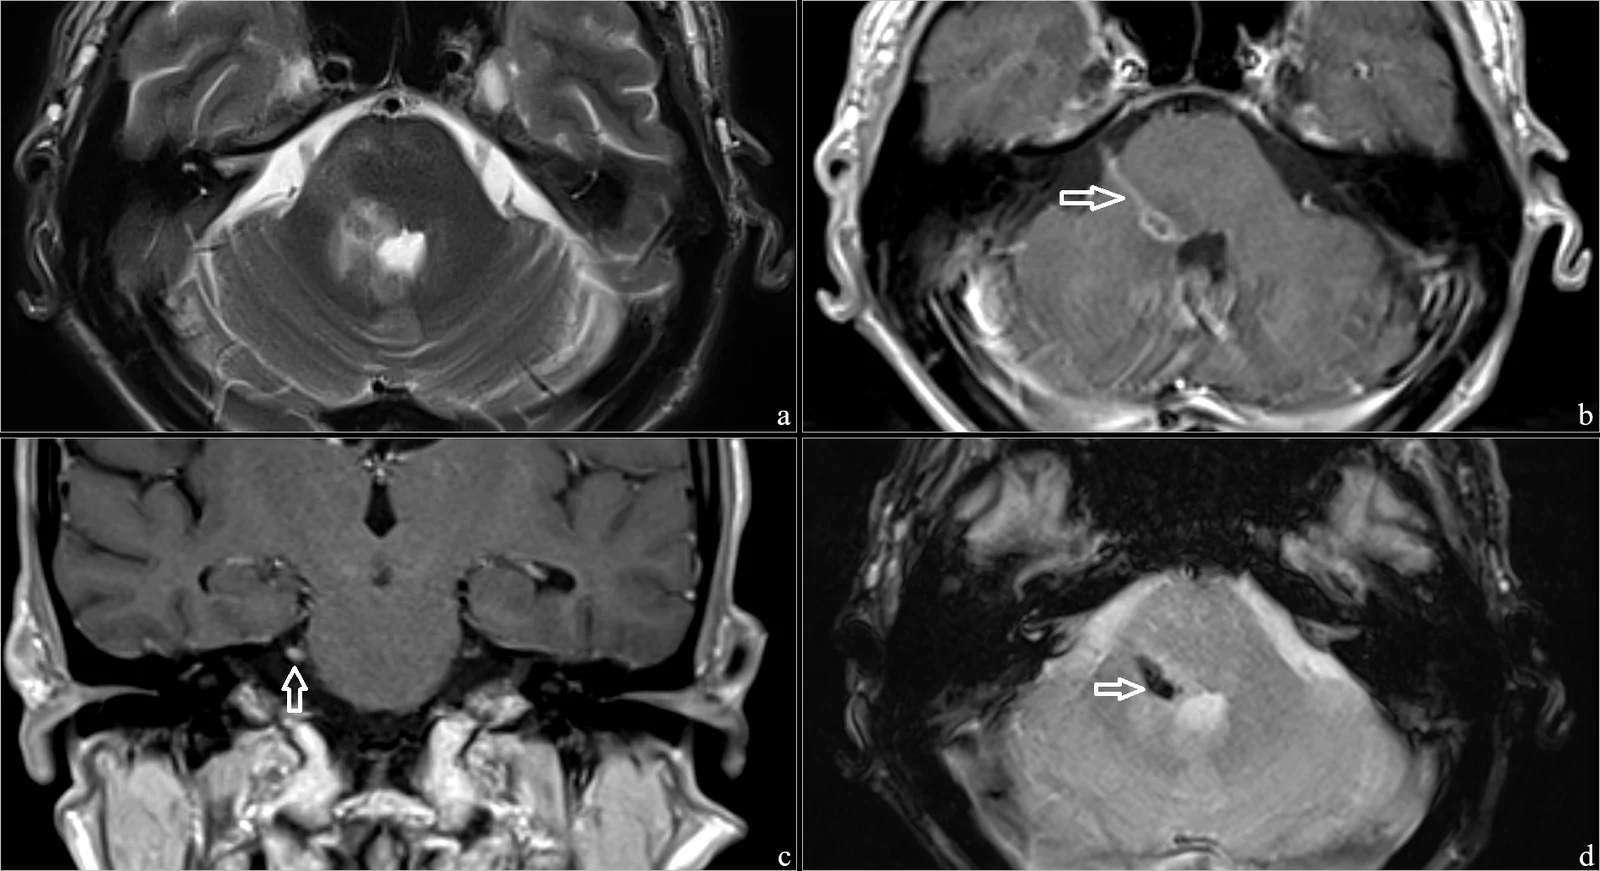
(a) T2-weighted sequence in the axial plane showing signal abnormalities in the right portion of the brainstem, specifically involving the lateral mixed system. (b) T1-weighted fat-suppressed contrast-enhanced sequence in the axial plane. (c) T1-weighted fat-suppressed contrast-enhanced sequence in the coronal plane both demonstrating significant involvement of the trigeminal nerve with possible involvement of its nuclei. (d) T2-weighted gradient-echo sequence in the axial plane suggesting a small hemorrhage extending from the pons to the right cerebellar peduncle at the midbrain–pons junction.
